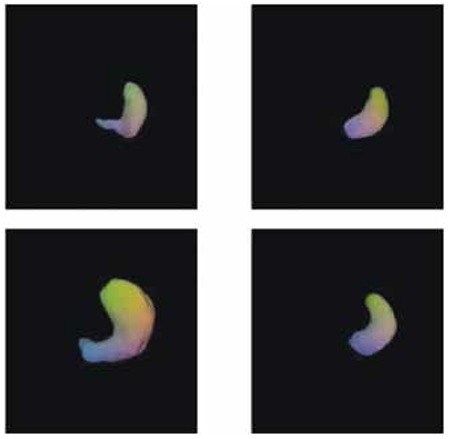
Examples of single photon emission computed tomography images of fasting gastric volumes (top row) and postprandial gastric volumes (botton row) in healthy individuals (left column) and patients with functional dyspepsia (right column)
